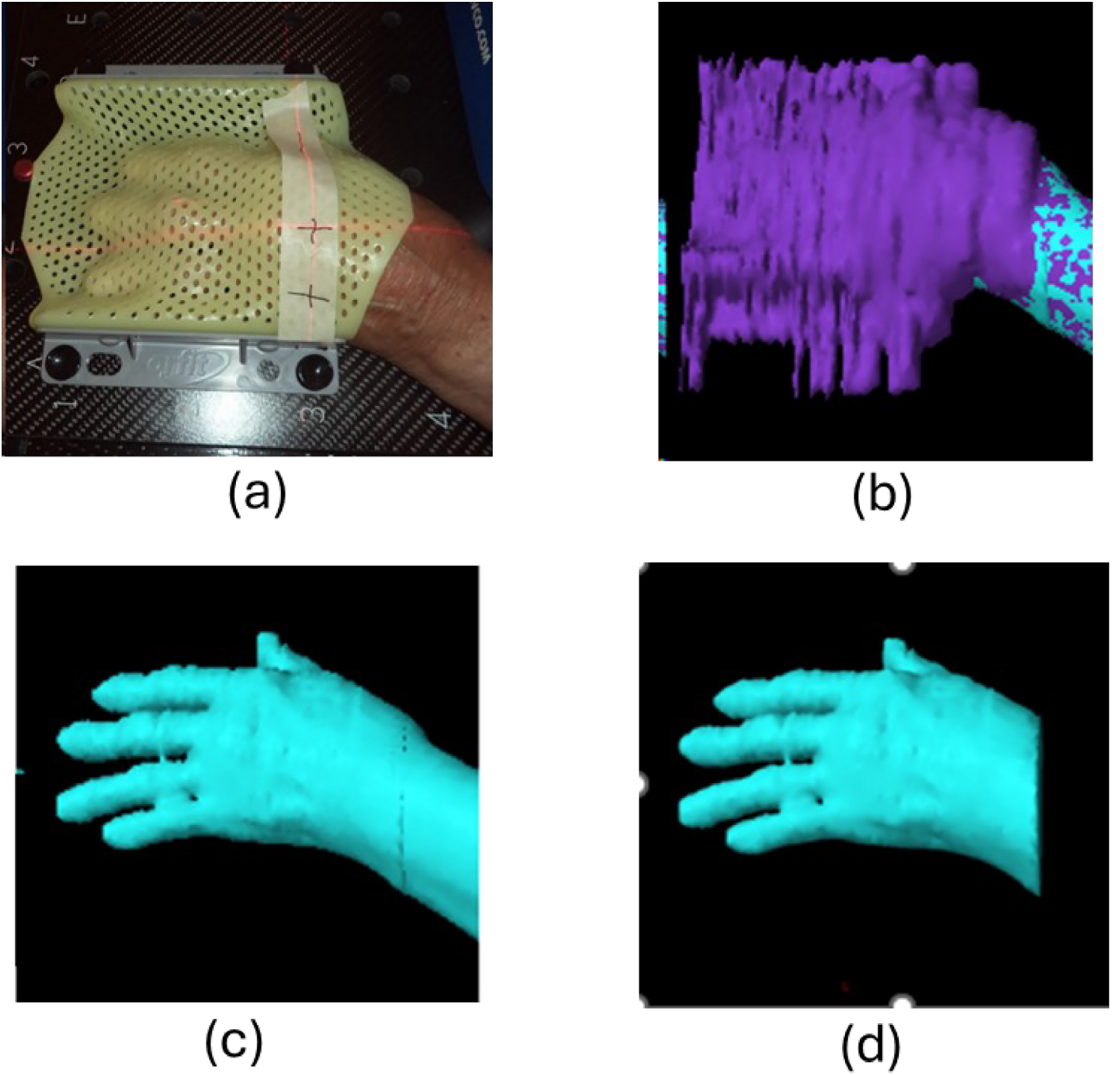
(a) photo of patient left hand CT Sim setup, (b) contour with mask, (c) left hand contour, and (d) target contour, which is in the primary beams.
